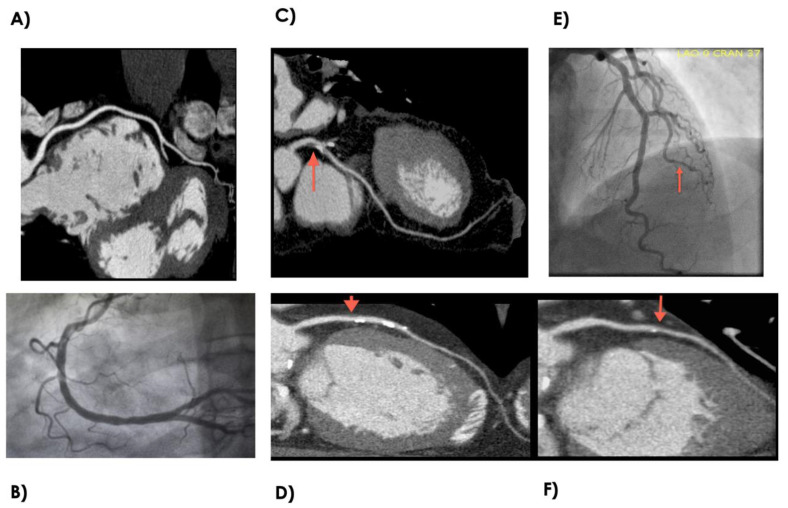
Example of normal and disease coronary anatomy as depicted by coronary CTA and coronary angiogram. (A) Normal right coronary artery by coronary CTA. (B) Normal right coronary artery by coronary angiogram. (C) Focal Mixed calcified atherosclerotic plaque in the proximal left anterior descending artery as depicted by coronary CTA. (D) Sequential soft lipid and calcified plaque in the mid-to-proximal left anterior decending artery as depicted by coronary CTA. (E) Luminal irregularities involving the 2nd diagonal branch as depicted by coronary angiogram. (F) Focal luminal remodeling with mix soft lipid plaque and spotty calcifications. CTA—Computed Tomography Angiography.
